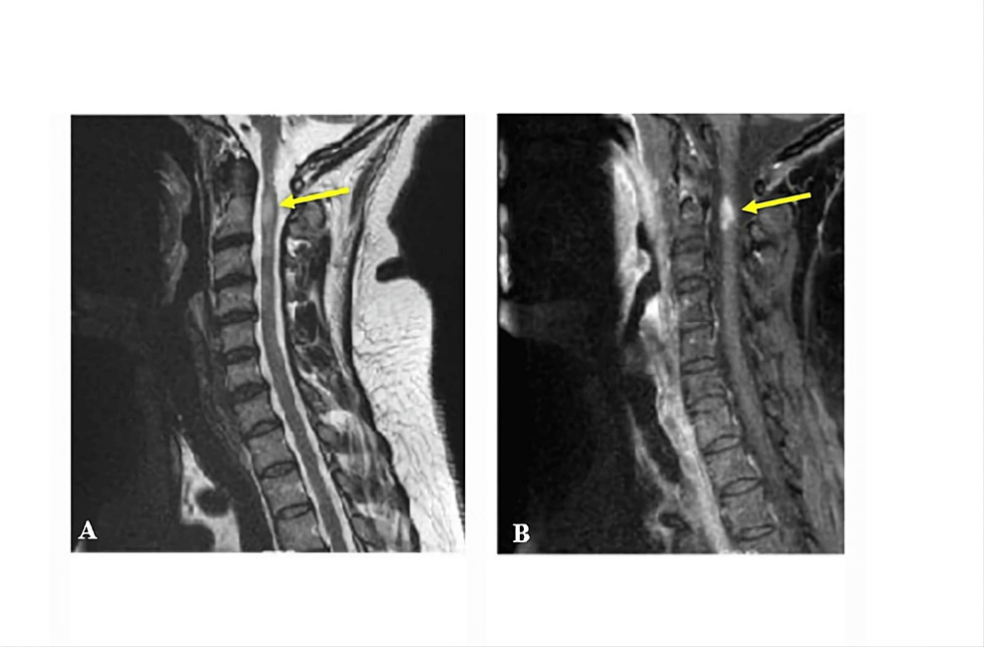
A 38-year-old female MS patient. (A) Sagittal T2-weighted imaging showing a bright signal intensity lesion in the cervicomedullary junction, left aspect of the cord opposite the C2 level. (B) T1-weighted imaging post-contrast administration demonstrating a intense enhancement lesion in the delayed phase.
